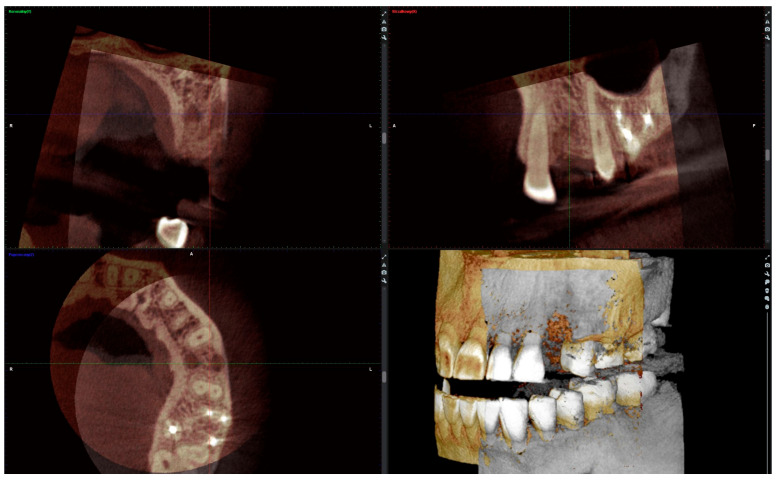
Superimposition of radiographic images.
